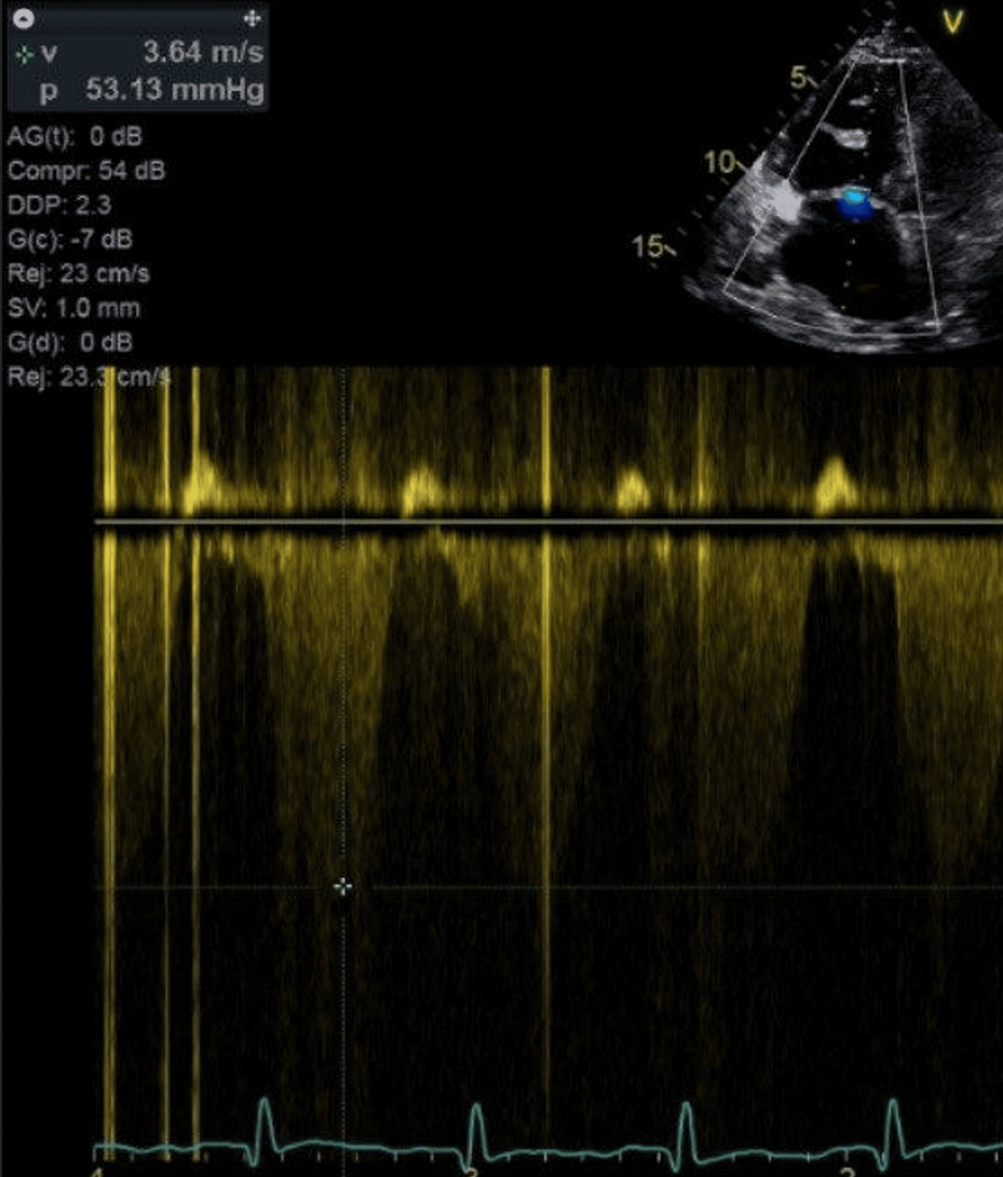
Doppler across the tricuspid valve showing TR with velocity of 3.6 m/sec, indicating an elevated pulmonary pressure. TR: tricuspid regurgitation
